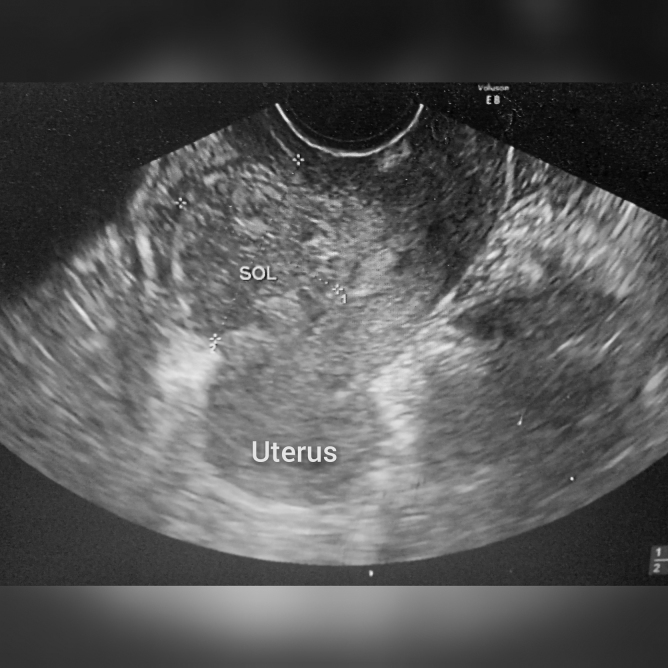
Transvaginal ultrasound image showing extrauterine heterogenous space occupying lesion communicating with the endometrial cavity.
